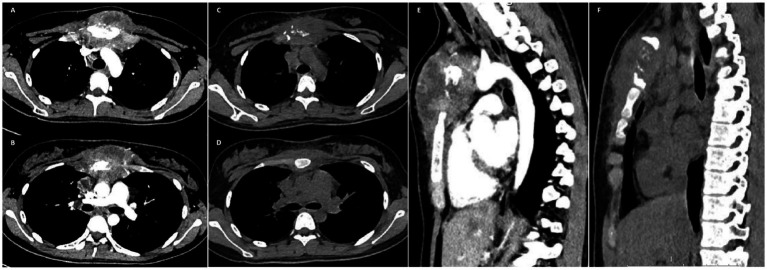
(A,B) Initial chest computed tomography (CT) revealed osteolytic destruction of the manubrium with associated soft tissue swelling. (C,D) Follow-up chest CT performed approximately 4 weeks after initiation of anti-tuberculosis therapy revealed a reduction in soft tissue swelling surrounding the manubrium. (E) Sagittal chest CT at baseline shows the sternal lesion. (F) Follow-up sagittal chest CT shows significant improvement of the sternal lesion compared to the baseline.
